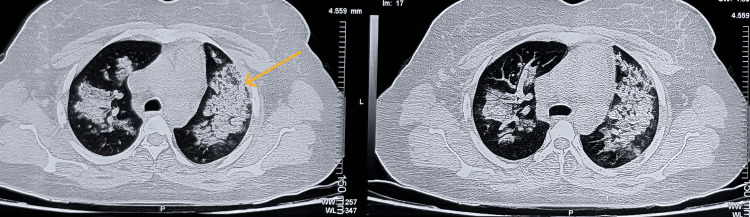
HRCT Thorax at admission showing diffuse alveolar hemorrhage. Extensive bilateral ground-glass opacities and alveolar infiltrates seen, consistent with acute diffuse alveolar hemorrhage. HRCT: high-resolution computed tomography
